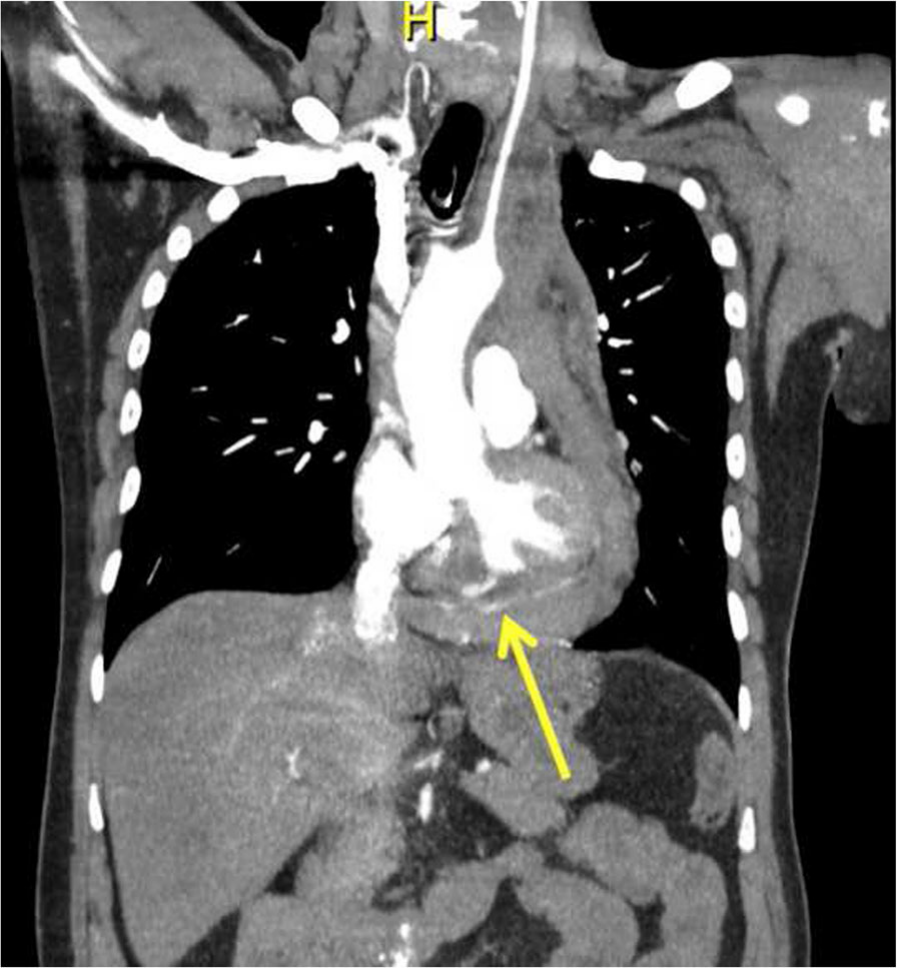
Coronal view of Aortic CTA revealing contrast leakage in the pericardium (yellow arrow) consistent with LVFWR
